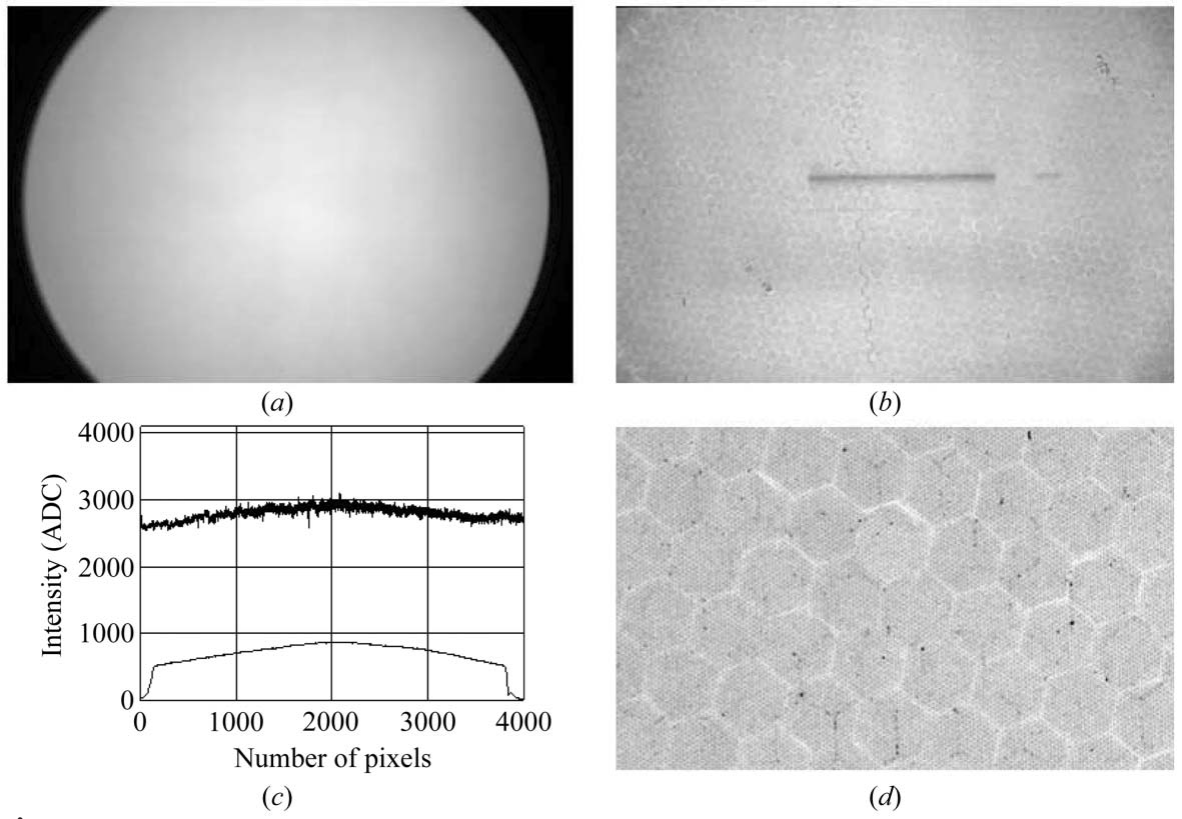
Uniformity of response. (*a*) A flat-field image recorded by the lens-coupled detector. The field of view is limited by the size of the phosphor, not by that of the CCD chip. (*b*) A flat-field image recorded by the fiber-coupled detector. The horizontally elongated area in the center with low intensity was caused by damage resulting from prolonged irradiation, most probably owing to browning of the optical fiber. These images were obtained with an X-ray energy of 23 keV. (*c*) Horizontal line profiles of the images at the center of images in (*a*) and (*b*). (*d*) Chicken-wire pattern in the fiber-coupled detector.
